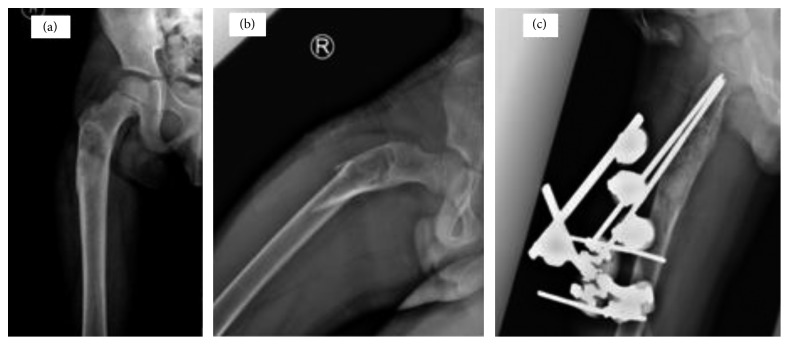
(a) AP Femur X-ray with proximal femur cyst diagnosed as benign fibrous histiocytoma. (b) The patient presented 2 years later with a pathological fracture. Final pathology revealed ABC. (c) Lateral X-ray of proximal femur with external fixator showing partial healing of the cyst and a well-maintained fracture reduction about 6 weeks after surgery.
